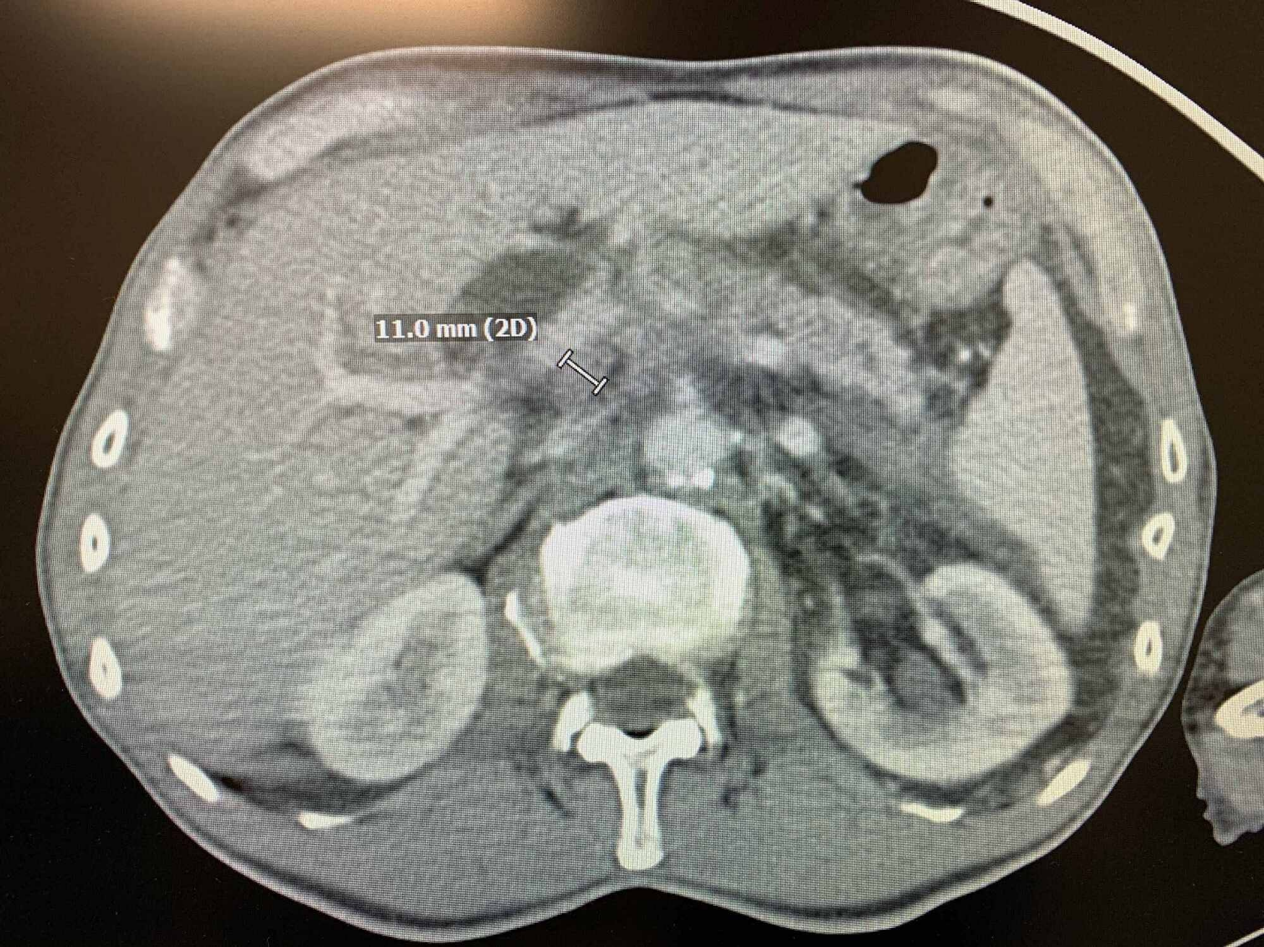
CT scan abdomen: CA pancreas Poorly delineated pancreatic head-neck junction mass measuring approximately 1.5 x 1 cm. There is ductal dilatation proximal to the mass up to 5 mm. Soft tissue density infiltration extending around the proximal portal vein, central superior mesenteric vein, central splenic vein, and proximal superior mesenteric artery is suspicious for locally advanced, unresectable disease. It also showed intra and extrahepatic biliary duct dilatation related to obstruction by the mass. CT: computed tomography; CA: Cancer
